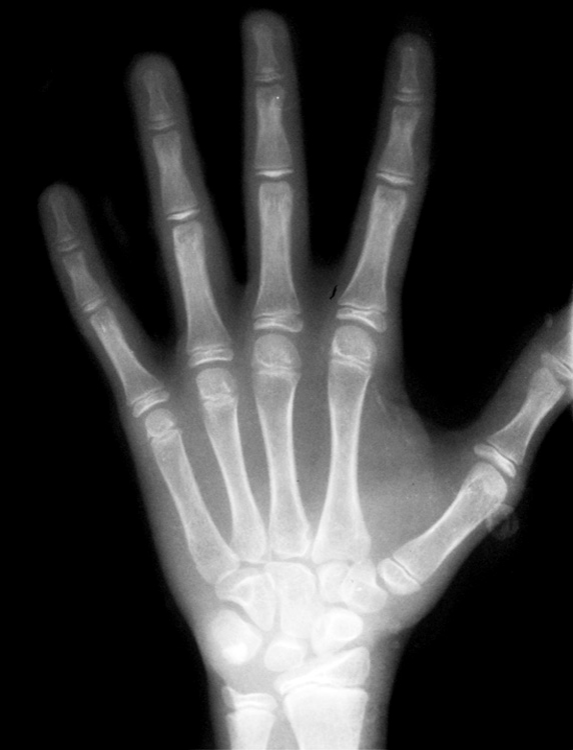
Anatomy of hand wrist radiographs
